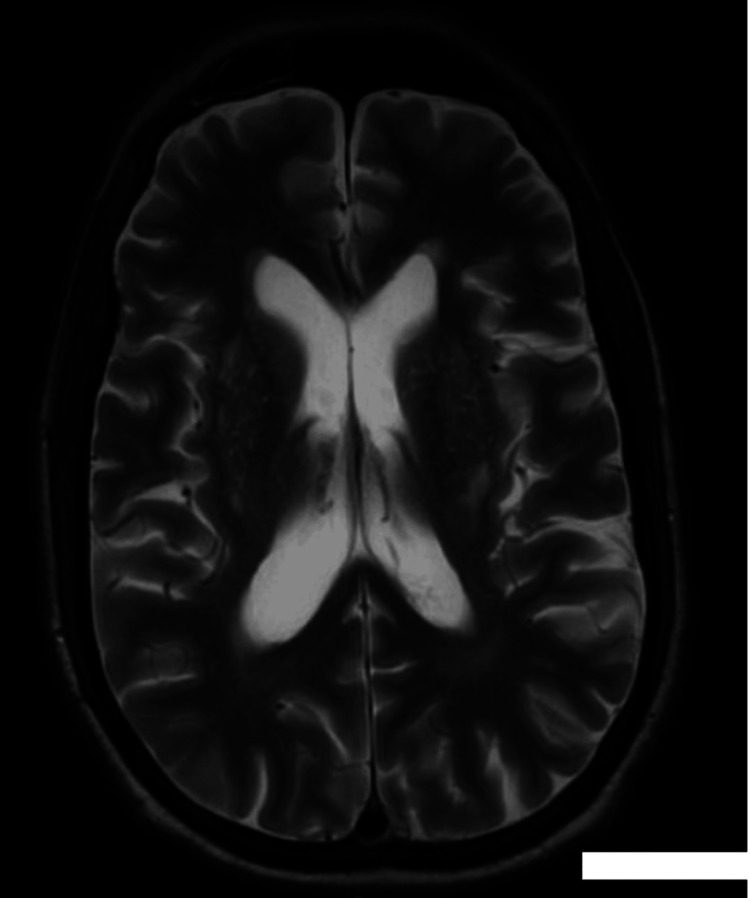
MRI brain - unremarkable.
